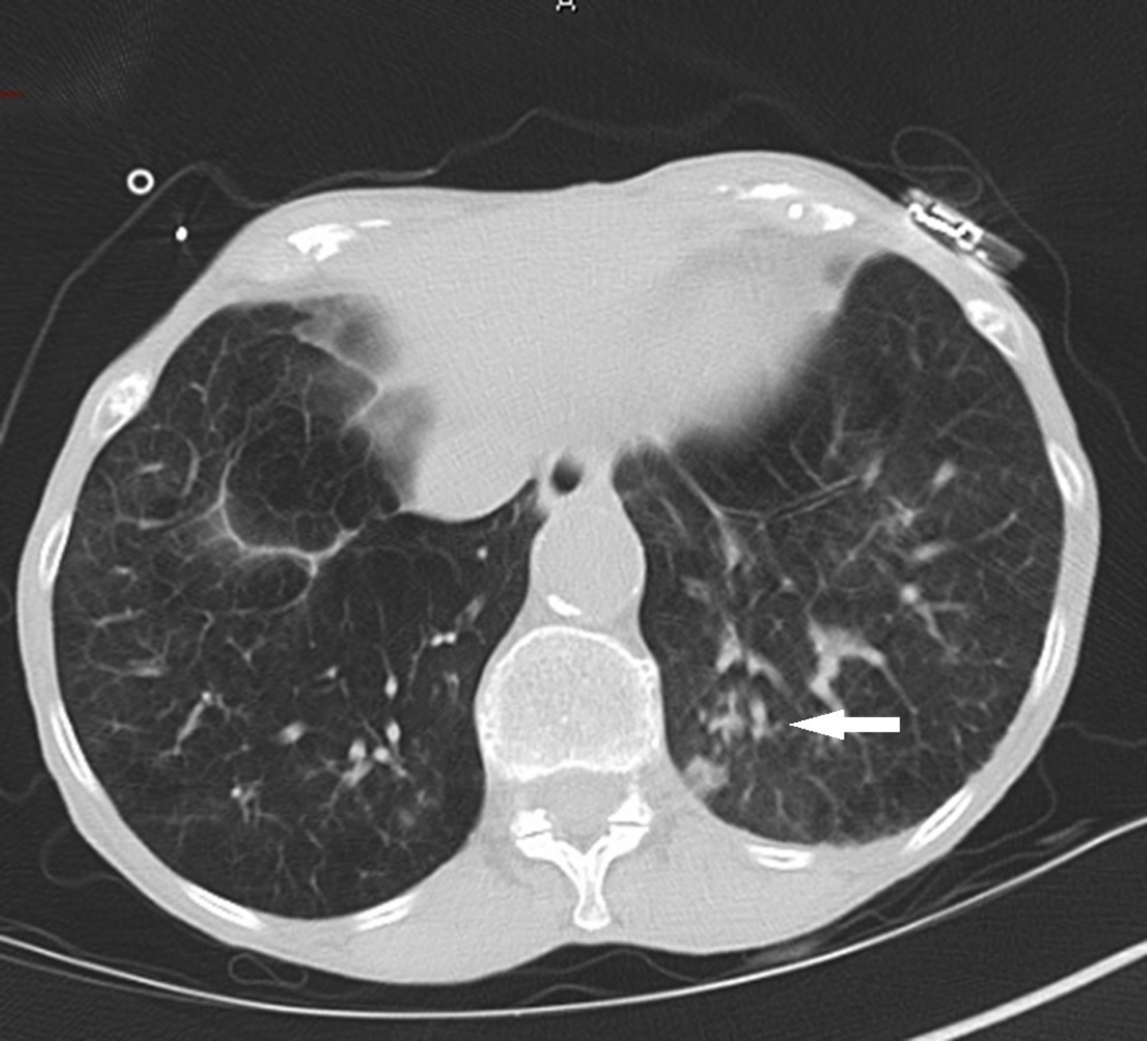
Computed tomography of the chest showed tree-in-bud opacities along with bronchial wall thickening (arrow) consistent with an infectious process.
